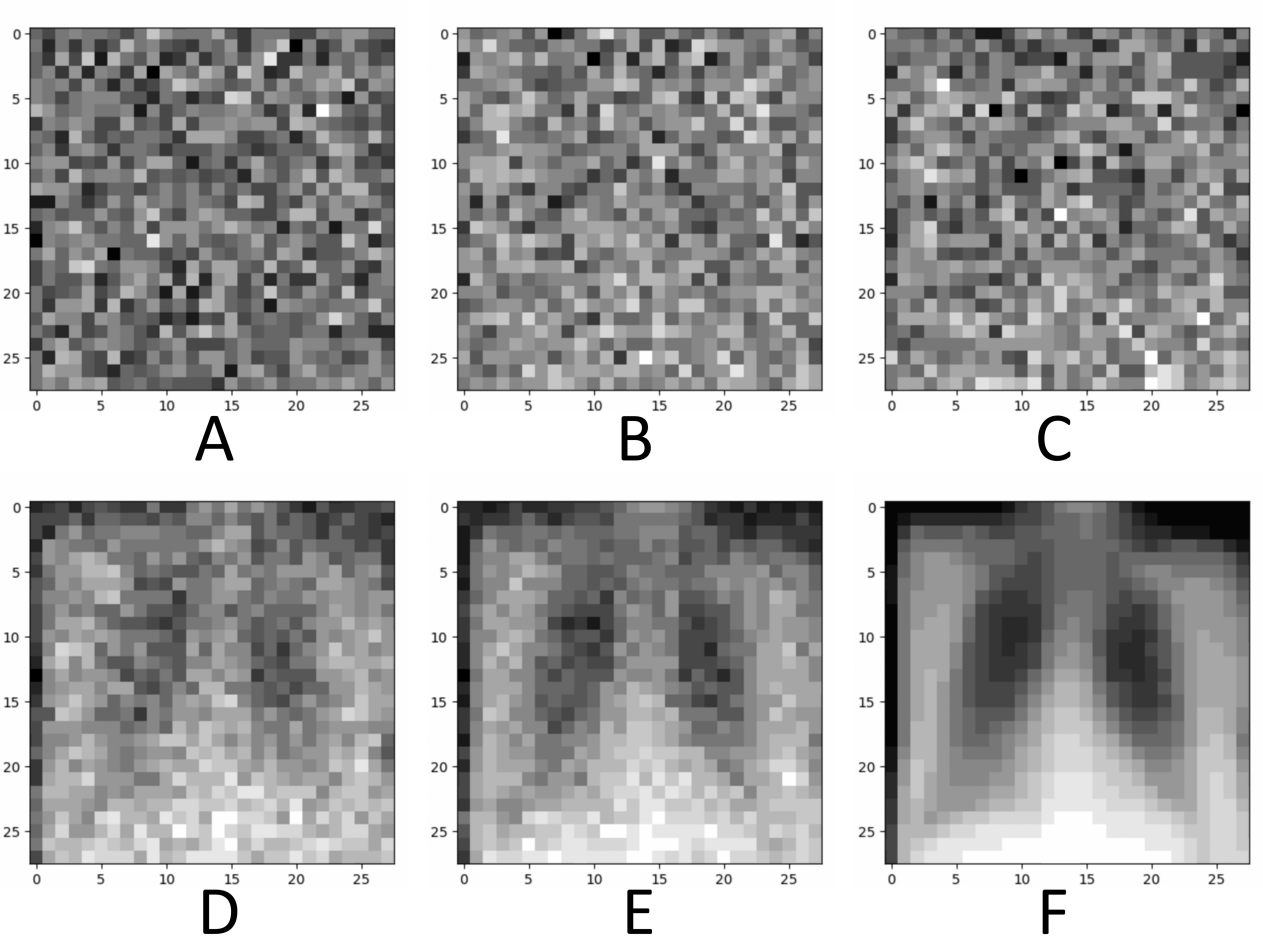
The process of generating an example chest X-ray image using the diffusion model. (A), The original Gaussian noise; (B), The image after 99 steps of denoising; (C), The image after 149 steps of denoising; (D), The image after 259 steps of denoising; (E), The image after 279 steps of denoising; (F), The final generated image after 299 steps of denoising.
